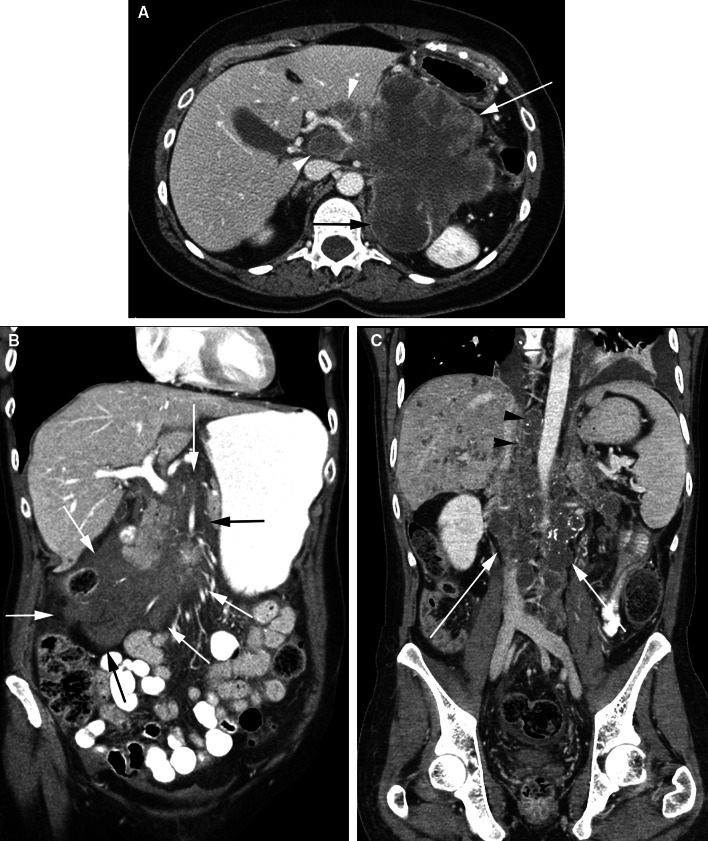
Subperitoneal spread of disease. A Axial CT image shows a large pancreatic mass (*white arrow*) extending along the hepatoduodenal ligament to the porta hepatis (*arrowheads*). Mass also invades the left perinephric space and engulfs the adrenal gland (*black arrow*). Superiorly, the mass extended into the gastrohepatic ligament with invasion of the left lobe of the liver (not shown). B Coronal CT image shows a hematoma in the root of the small bowel mesentery extending toward the ileocecal junction within the small intestine mesentery (*arrows*). C Coronal CT image shows a heterogeneous mass (*white arrows*) around the inferior vena cava and aorta. Mass extends superiorly, through the aortic hiatus, to the posterior mediastinum (*black arrowheads*). The mass also extended into the root of the small bowel mesentery (not shown) along the superior mesenteric artery.
